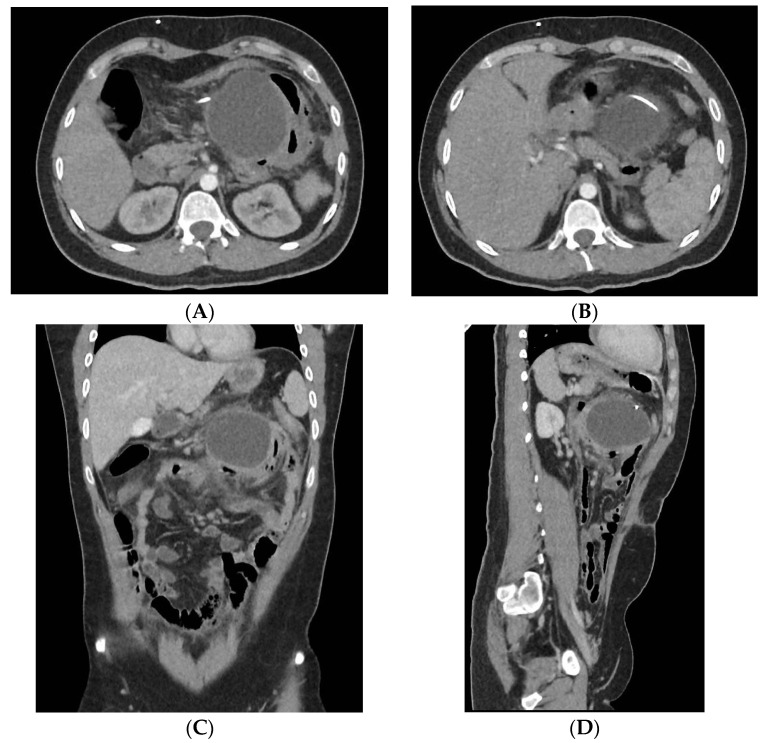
Abdominal Computer Tomography (CT) scan sections reveal a CSF pseudocyst—a well-defined liquid collection, round in shape, with axial dimensions of approximately 77 mm. Axial planes (A,B), coronal plane (C), and sagittal plane (D) of the CT scan.
